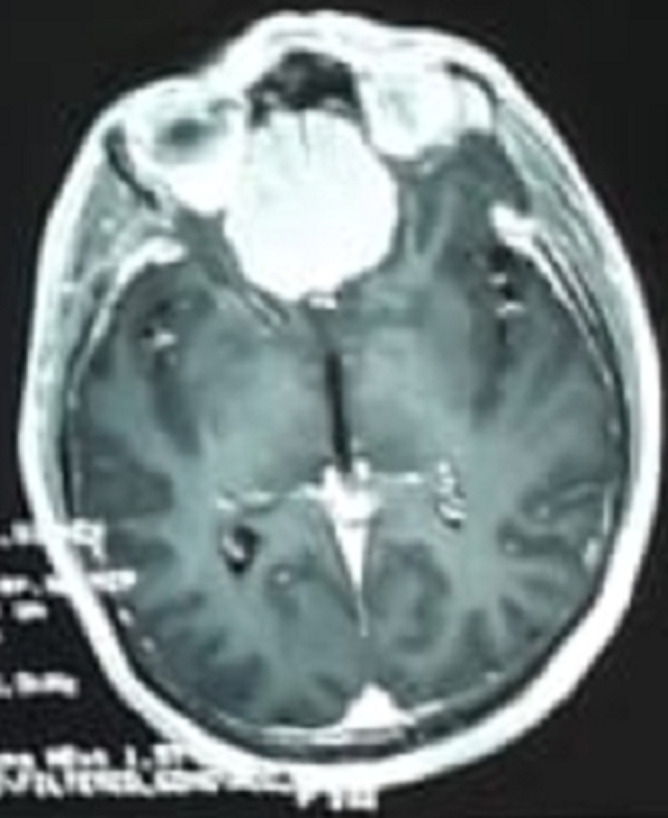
MRI scan of T1 lesion hypo intensity extra axial anterior skull base fronto ethmoidal area; in T2 lesion hyper intensity this lesion was homogenous inhancing after gadolinium injection
